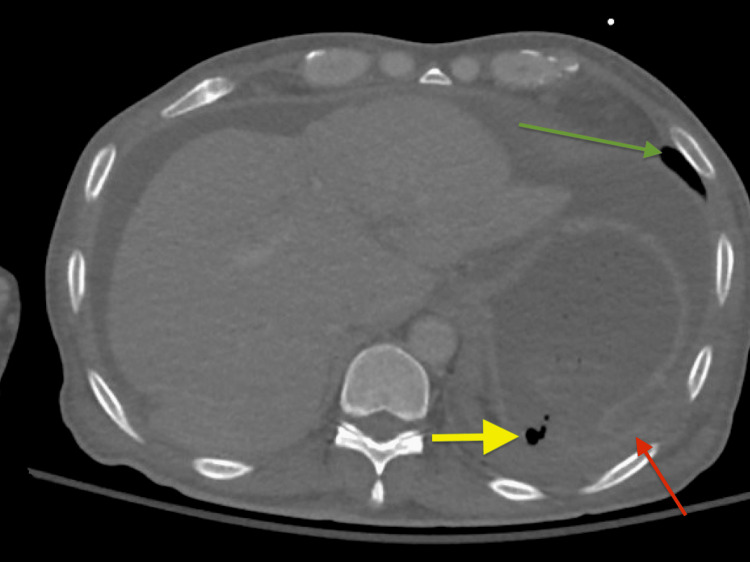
CT chest, showing left diaphragmatic interruption with herniation of gastric fundus into the left hemithorax. Yellow arrow: Foci of air adjacent to the gastric fundus, concerning for gastric rupture, Red arrow: complex left pleural effusion, Green arrow: small-to-moderate volume left-sided pneumothorax.
